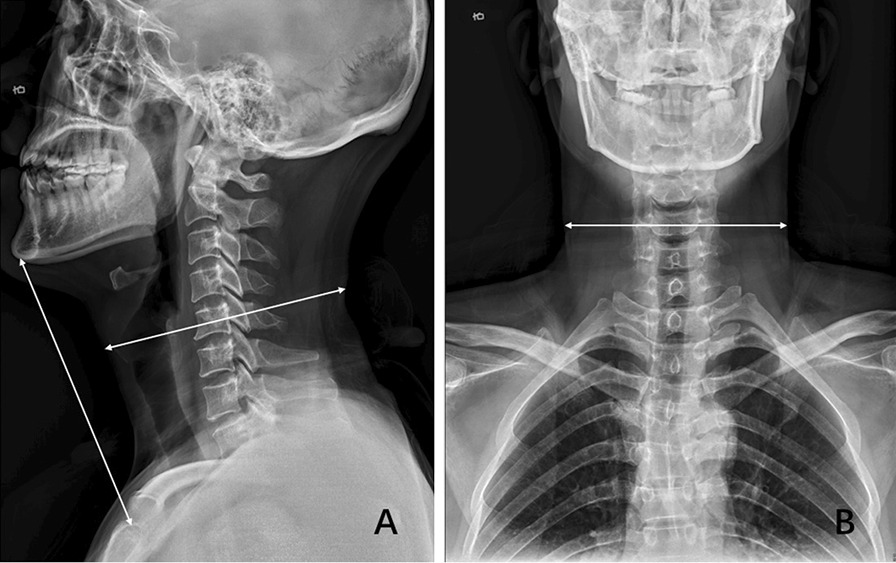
Sagittal radiograph showing that the cervical length was measured as the distance between the sternum and gnathion. Sagittal and coronal cervical circumferences were defined as the distance between the intersection of the line parallel to the endplate through the midpoint of C5 and soft tissue shadow
